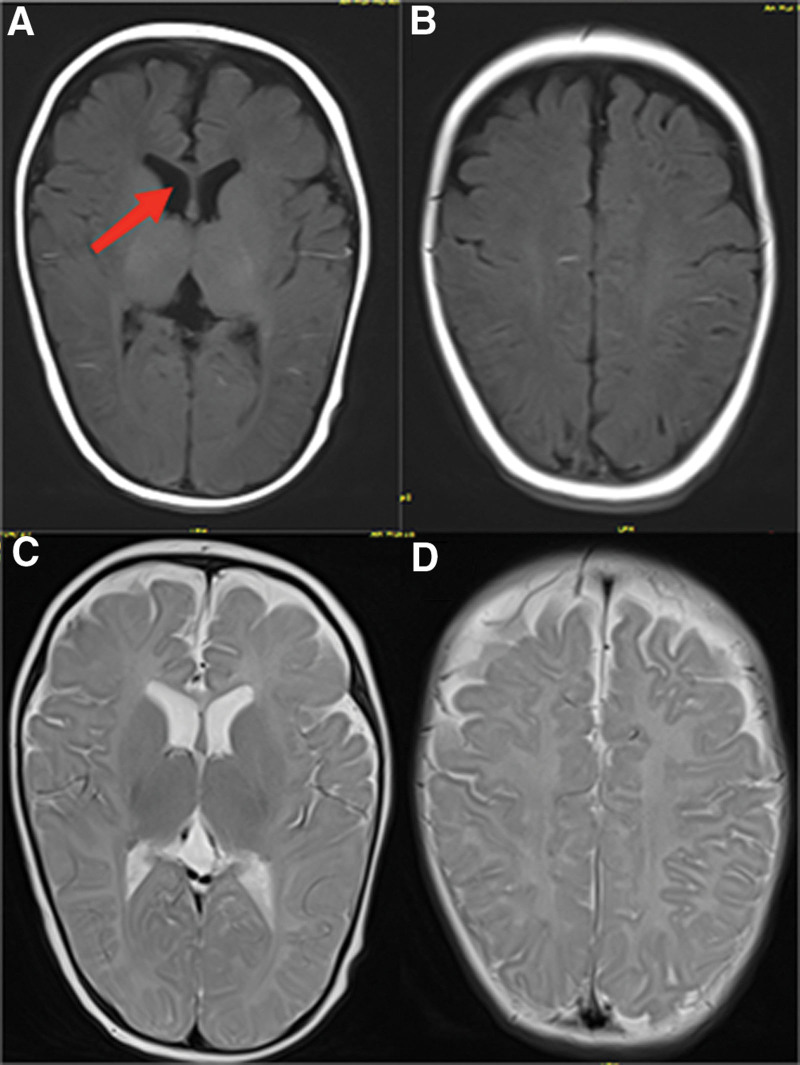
Brain MRI performed at five and a half months of age. Axial T1-weighted image shows no high signal in the knees of the corpus callosum, as represented by red arrows, which indicates delayed myelination. Decreased brain volume was observed simultaneously in T1-weighted (A and B) and T2-weighted images (C and D).
